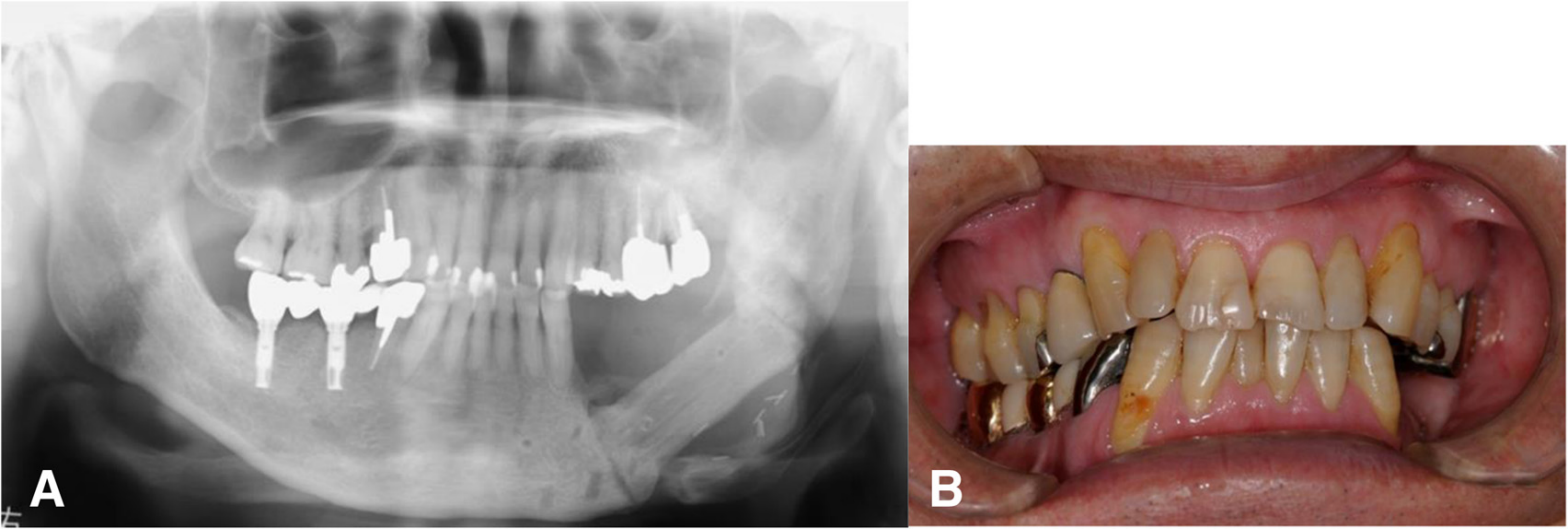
a Panoramic X-ray image 1 year after the surgery. b Intraoral photo 1 year after the surgery
